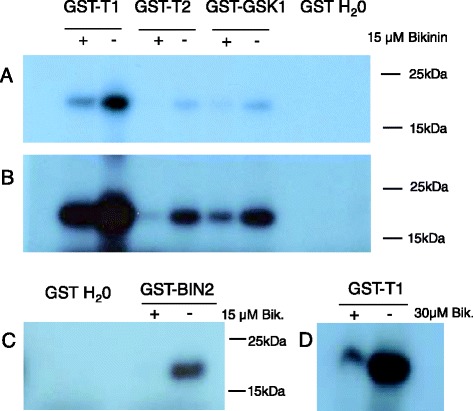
In vitro* kinase activity of TaSKs in the presence of Bikinin. Phosphorylation activity of purified TaSK1, TaSK2, TaGSK1 and BIN2 on a myelin basic protein fragment (18.454 kDa) was determined by *in vitro* kinase assays using ATP γP^32^. Kinase activity was analyzed in the presence and absence of Bikinin added at a concentration of 15 or 30 μM. After kinase reaction, samples were loaded on a 12 % SDS PAGE gel. After migration, the gel was directly exposed to an X-ray film for either 80 min at room temperature (a), o/n at room temperature (b), o/n at -80 °C (c), o/n at room temperature (d)
